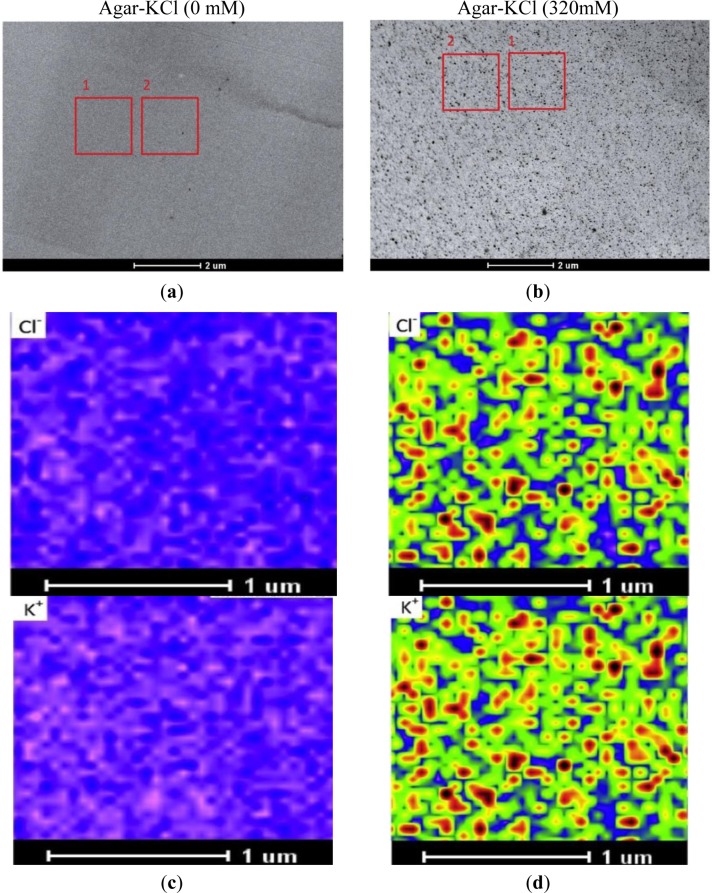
Representative STEM images (a,b) and elemental images (c,d) of 5% agar containing 0 or 320 mM KCl. STEM images with frame 1 showing the area for drift correction and frame 2 the measuring area. Elemental images of X-ray intensities with the scale fixed between the maximum (black) and minimum pixel intensities (violet) in a range of 25–1000 for Cl^−^ and 20–1000 for K^+^.
